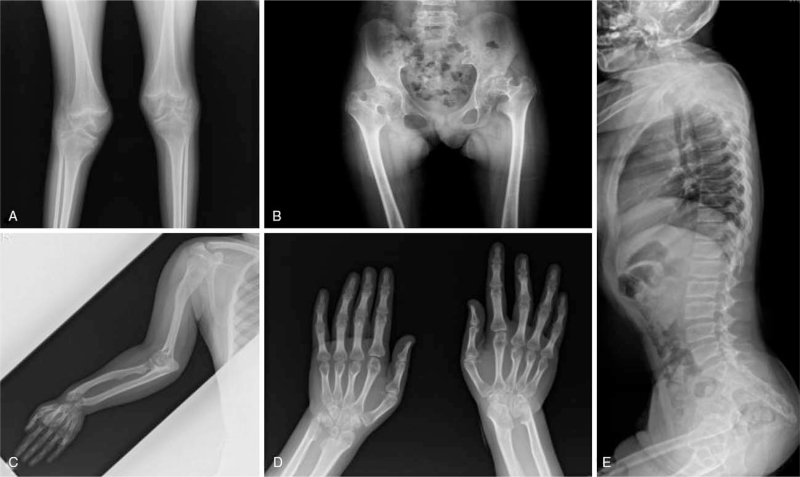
Knee and pelvic radiographs (A, B: patient's age:11 years old) and Right upper extremities, hands, spine lateral radiographs (C, D, E: patient's age: 23 years old). (A and B) Diffuse bony irregularity in articular surface of both knee. Flattened femoral head and articular surface, mild subluxation on the left superior femoral head. Secondary osteoarthritic change at both hip and knee joints. (C) Right upper extremity X-ray showed bowing deformities of both humerus, radius, and ulna. Both humeral heads showed deformity due to epiphyseal dysplasia. (D) Carpal bones and the distal epiphysis of both radius and ulna showed deformity. (E) Spine lateral X-ray showed congenital vertebral block in C3–C5.
